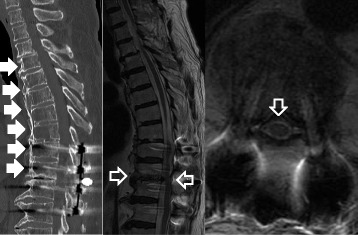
Postoperative CT revealed diffuse idiopathic skeletal hyperostosis between T4 and T10 (*white solid arrows*). Postoperative MRI revealed that spinal cord compression was well decompressed and high signal intensity in T2-weighted image was improved (*white outline arrows*)
